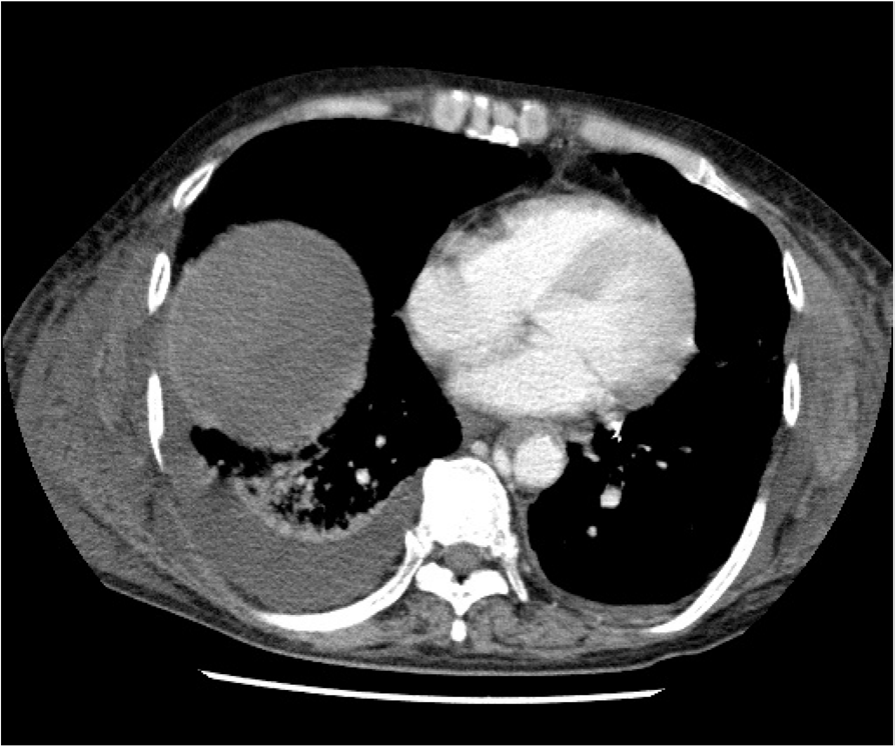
CT on the 5th POD revealed a dissection of the thoracic aortic aneurysm
